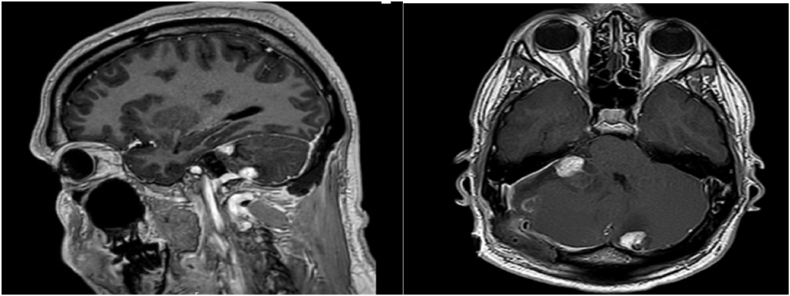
Orbital-brain MRI reveals multiple, non-expanding, centimetric nodular signal abnormalities of the subcortical white matter unaltered after contrast injection.
